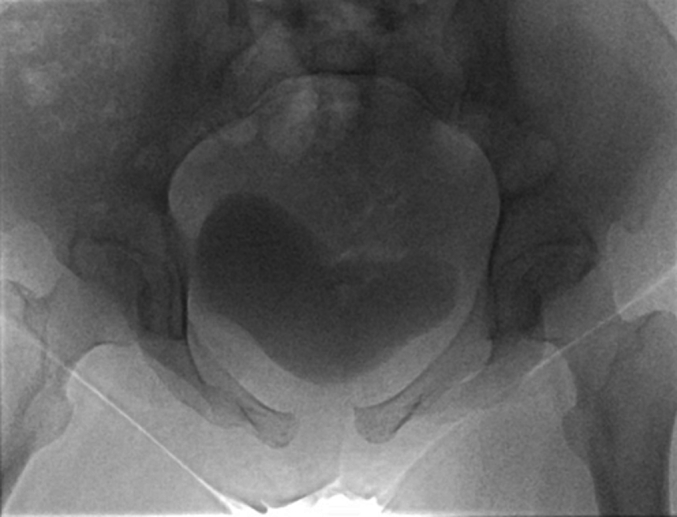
Fluoroscopic stress radiograph.
